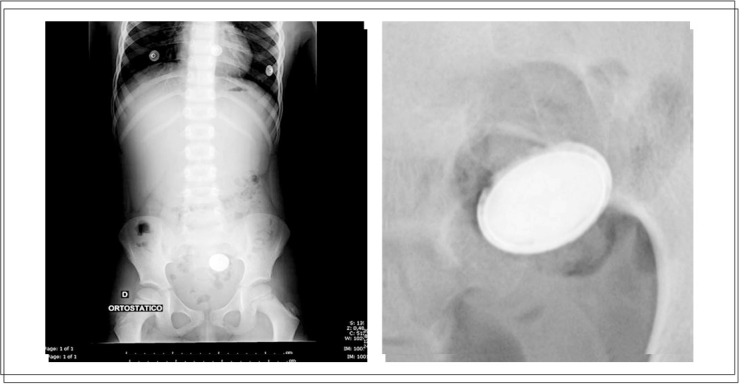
Simple abdominal radiography showed a radiopaque image with double circular halo outline in the distal colon.
